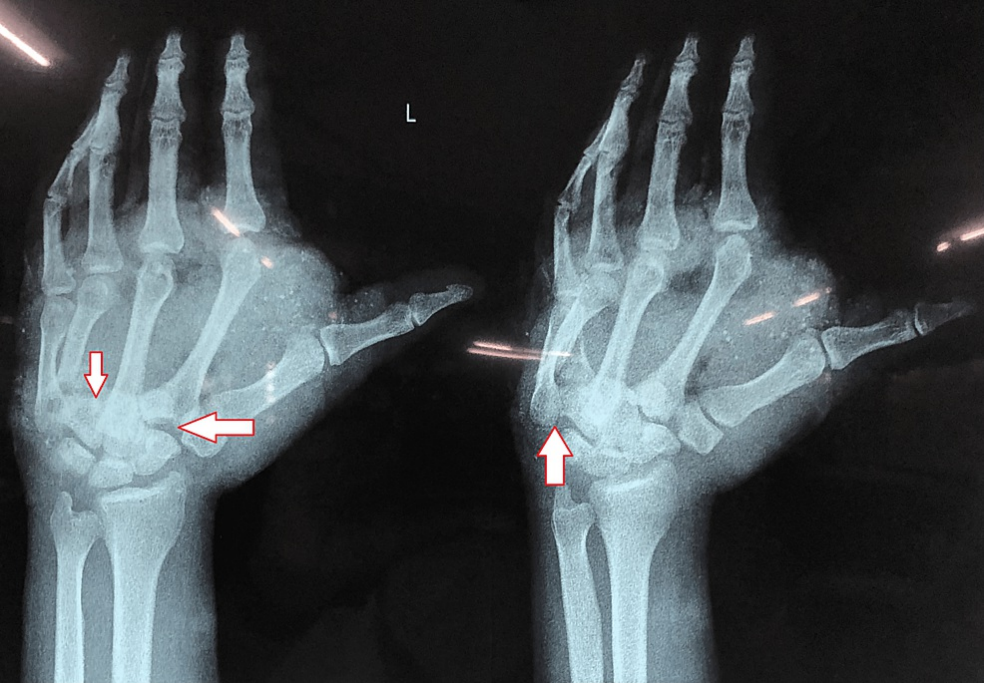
Pre-operative anteroposterior view of X-ray The image on the left shows left-hand fracture dislocation of the second, third, fourth, and fifth carpometacarpal joints. The image on the right indicated fracture of the base of fifth metacarpal.
